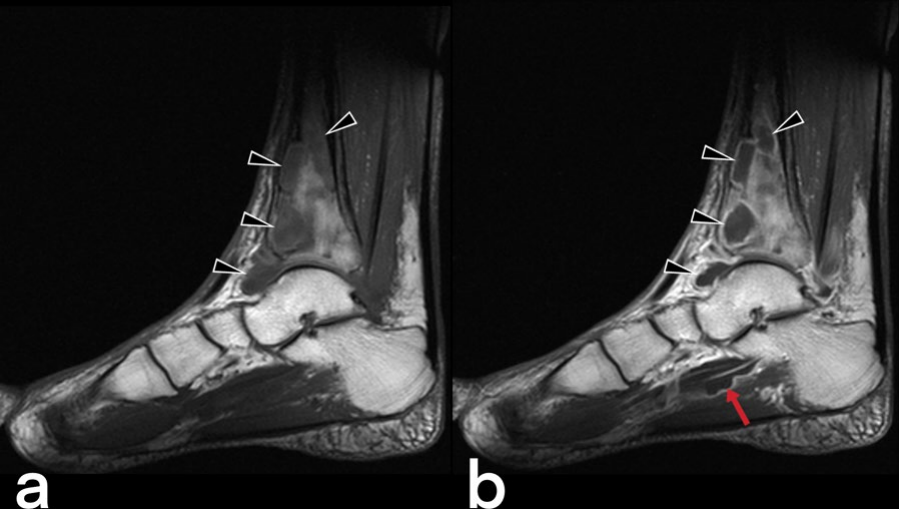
MR images of a 59-year-old male. Pre-contrast (a) and postcontrast (b) T1 weighted images demonstrate intraosseous (arrowheads) and soft tissue abscesses (arrow) in the distal part of the tibia and plantar face of the foot
